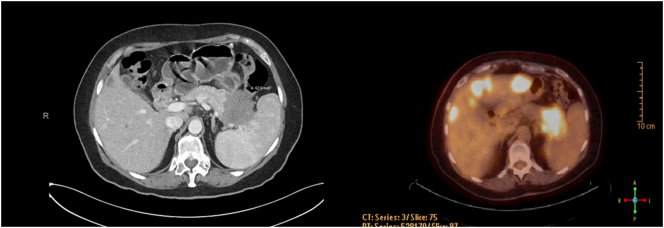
Pancreatic Tail lesion (CT with contrast and PET/CT, side by side). The prominent pancreatic tail mass invades into the splenic hilum, stomach, and splenic flexure, encasing the splenic vessels.
